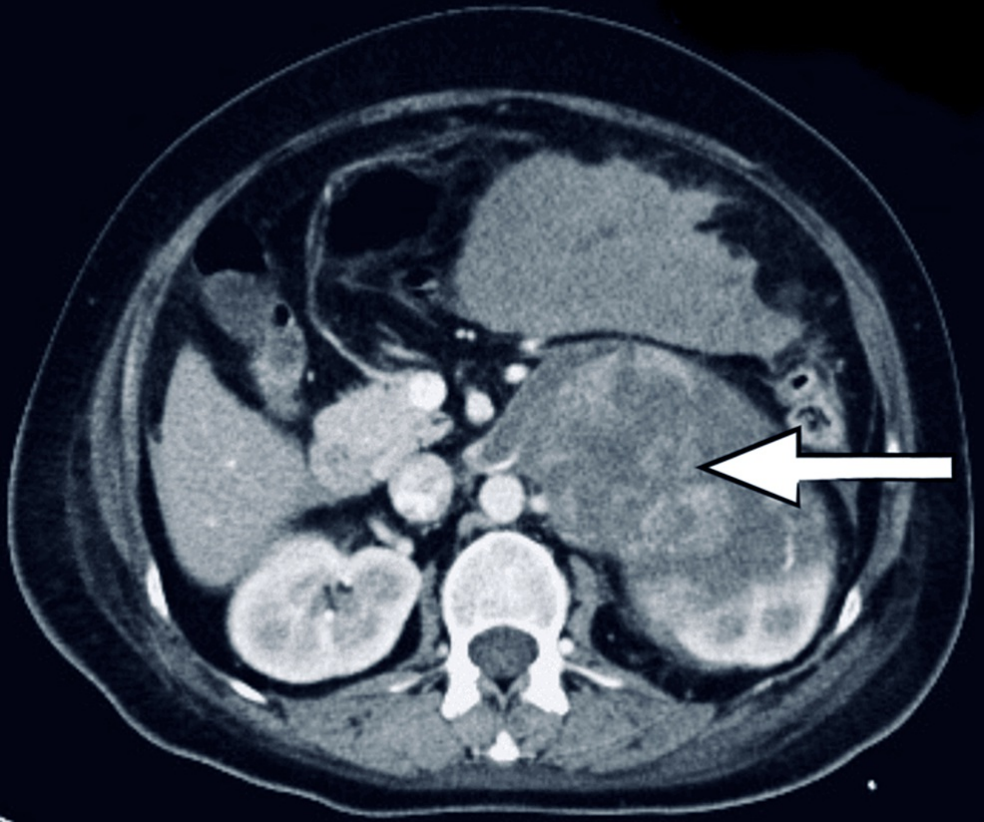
Contrast-enhanced CT abdomen (axial section) showing a large heterogeneously enhancing left adrenal mass with ?rupture with hemoperitoneum (solid white arrow).
